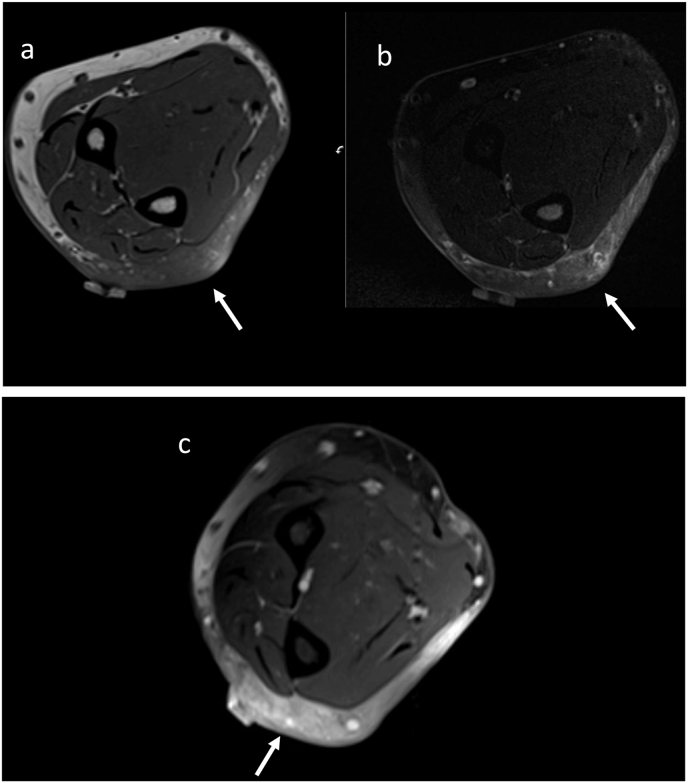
a, b, c: (a) Axial T1-weighted MRI image demonstrates diffuse low signal non mass like infiltration of the subcutaneous tissues of the posterior elbow (arrow). (b) Axial fat saturated T2 image shows areas of increased reticular signal within the posterior elbow subcutaneous tissues (arrow). (c) Axial contrast enhanced fat-saturated T1-weighted MRI image demonstrates diffuse non mass like enhancement of the subcutaneous tissues of the posterior elbow (arrow).
